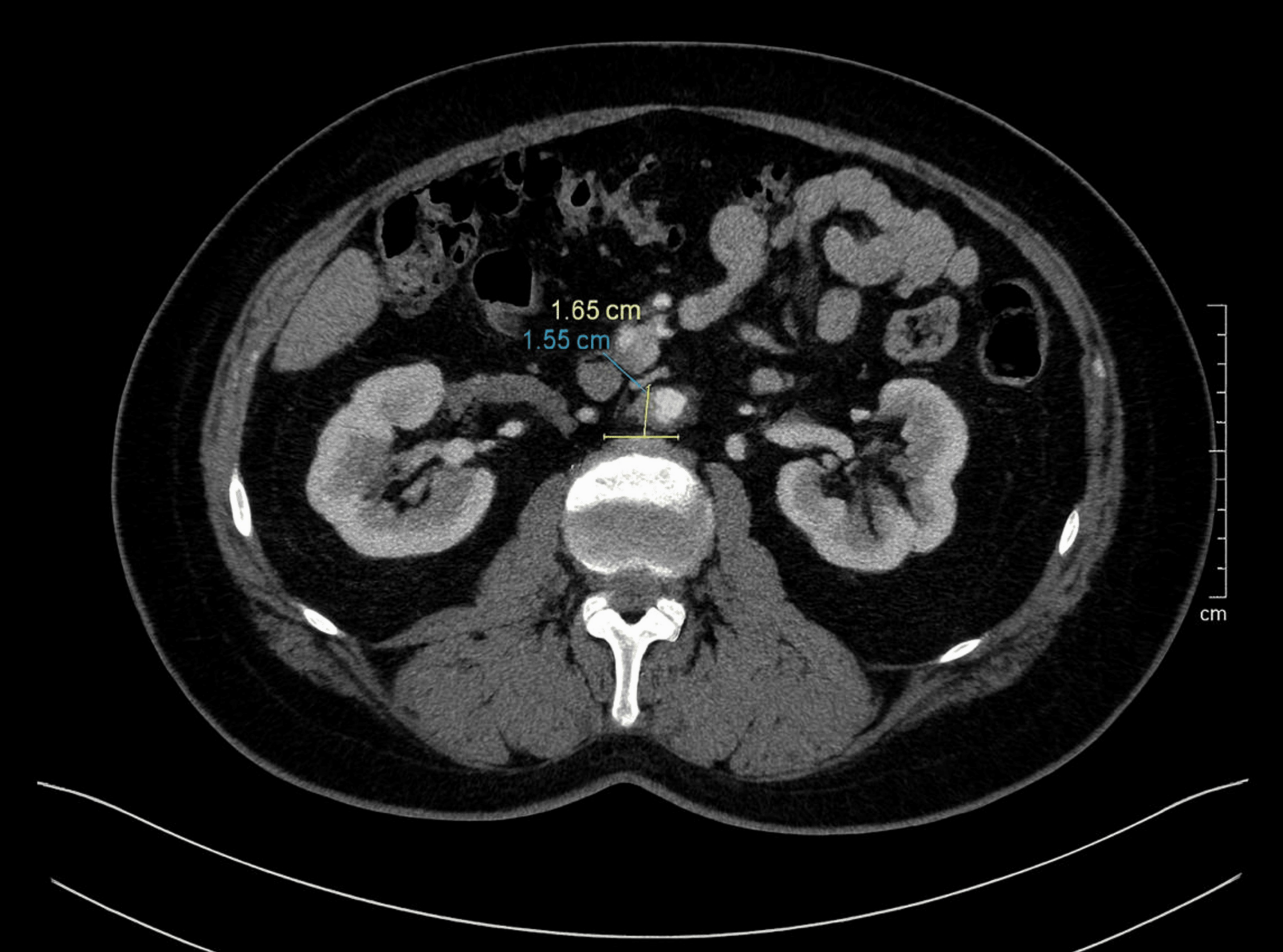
Six-year follow-up CT scan CT scan from January 2025, six years post-SBRT, showing continued tumor shrinkage with a residual lesion measuring 1.7 × 1.6 cm. CT: Computed tomography; SBRT: Stereotactic body radiation therapy
